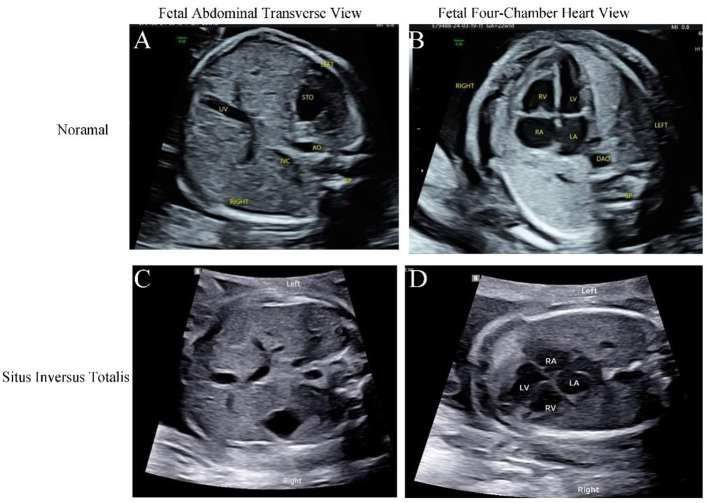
Ultrasonography of a normal fetus: transverse abdominal view (A) and four-chamber view of the heart (B); Ultrasonography of fetal situs inversus (C, D). (C) Transverse abdominal view showed the stomach bubble located in the right abdomen, the liver in the left abdomen, the abdominal aorta situated right anterior to the spine, and the inferior vena cava located left anterior to the abdominal aorta. (D) Four-chamber view showed the heart positioned in the right hemithorax with atrial inversion, right-looping ventricle, and discordant atrioventricular connection.
